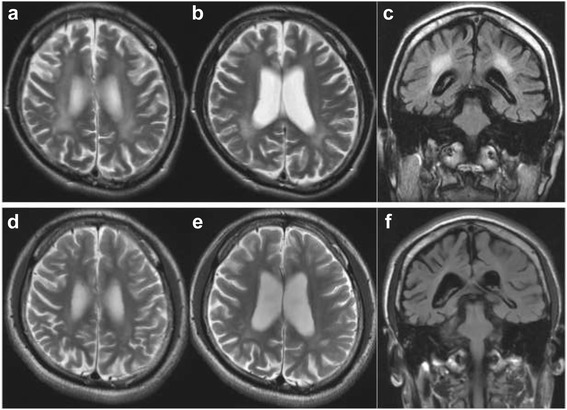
a-c Axial and coronal T2 weighted magnetic resonance imaging (MRI) images and axial fluid-attenuated inversion recovery (FLAIR) MRI image obtained at age 15 reveal a high-intensity area in the white matter around the posterior horns of the lateral ventricles. d-f Axial and coronal T2 weighted MRI images and FLAIR MRI image obtained at age 16 (8 months after betaine treatment) show resolution of the hyper-intensity in the white matter
